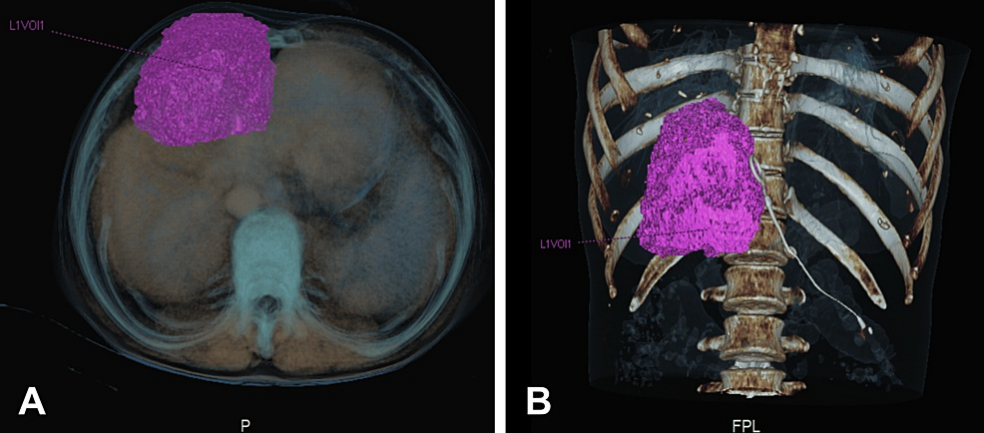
Abdominal CT three-dimensional reconstruction. Hypodense liver mass inside the HCC exhibiting an augmented peripheral rim or capsule (pink mass marked as L1VOI1). A: Transverse cut. B: Coronal cut. HCC: Hepatocellular carcinoma
